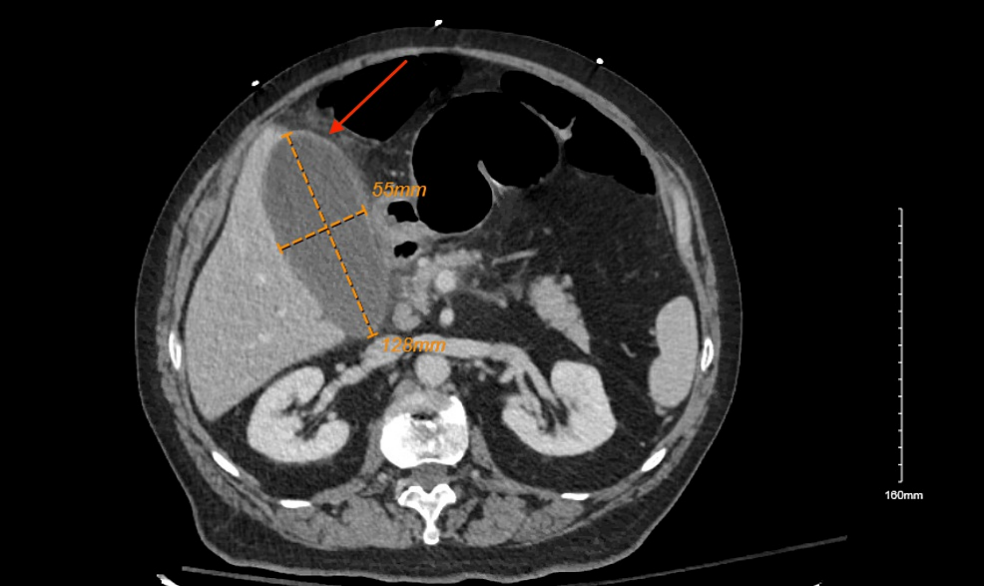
CT of the abdomen and pelvis demonstrating the size of the gallbladder and the red arrow indicating stranding surrounding the gallbladder CT: computed tomography
